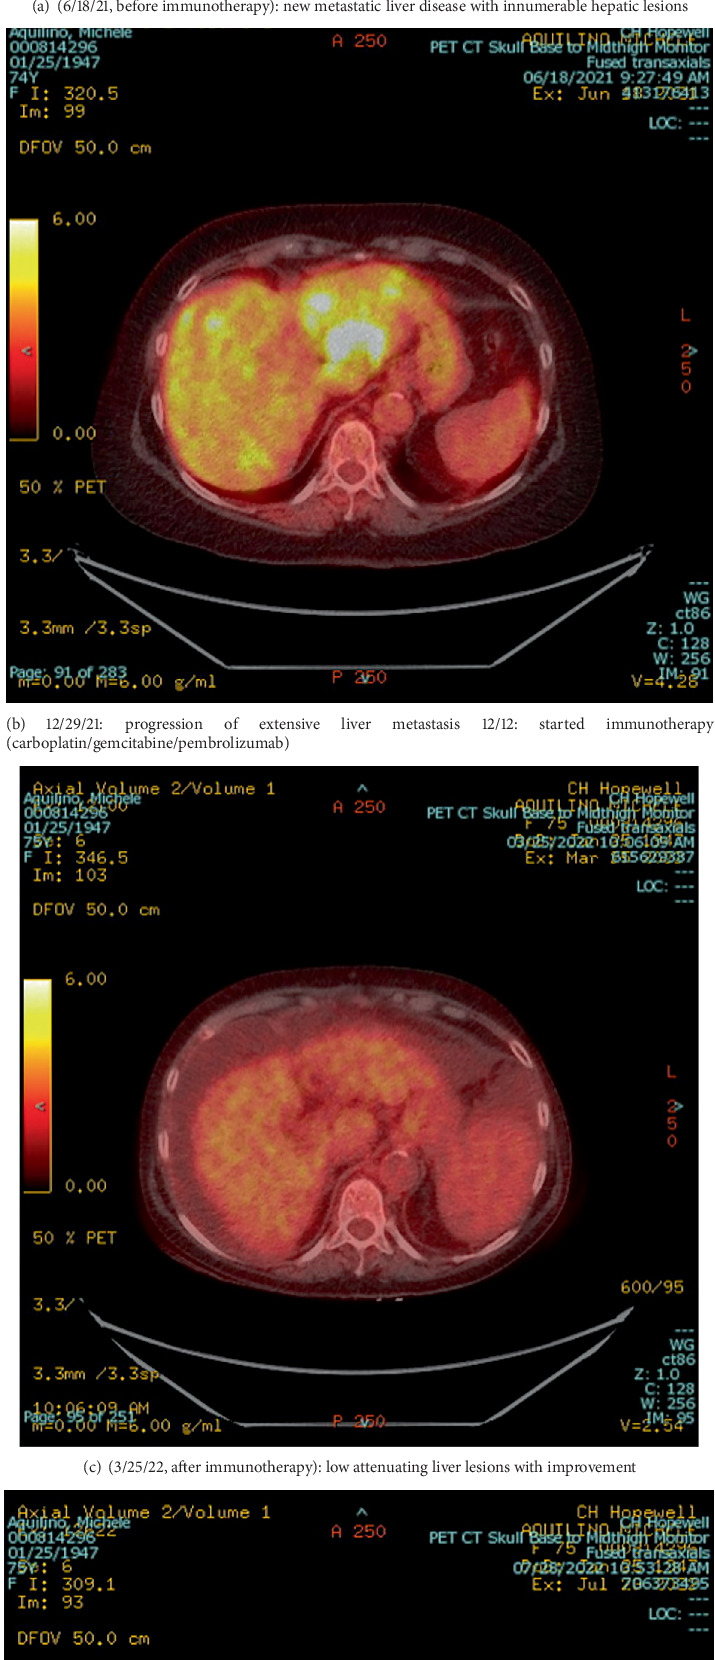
(a–d) Follow-up PET scan imaging of liver metastasis lesions at different stages of treatment. FDG, F-fluorodeoxyglucose.
